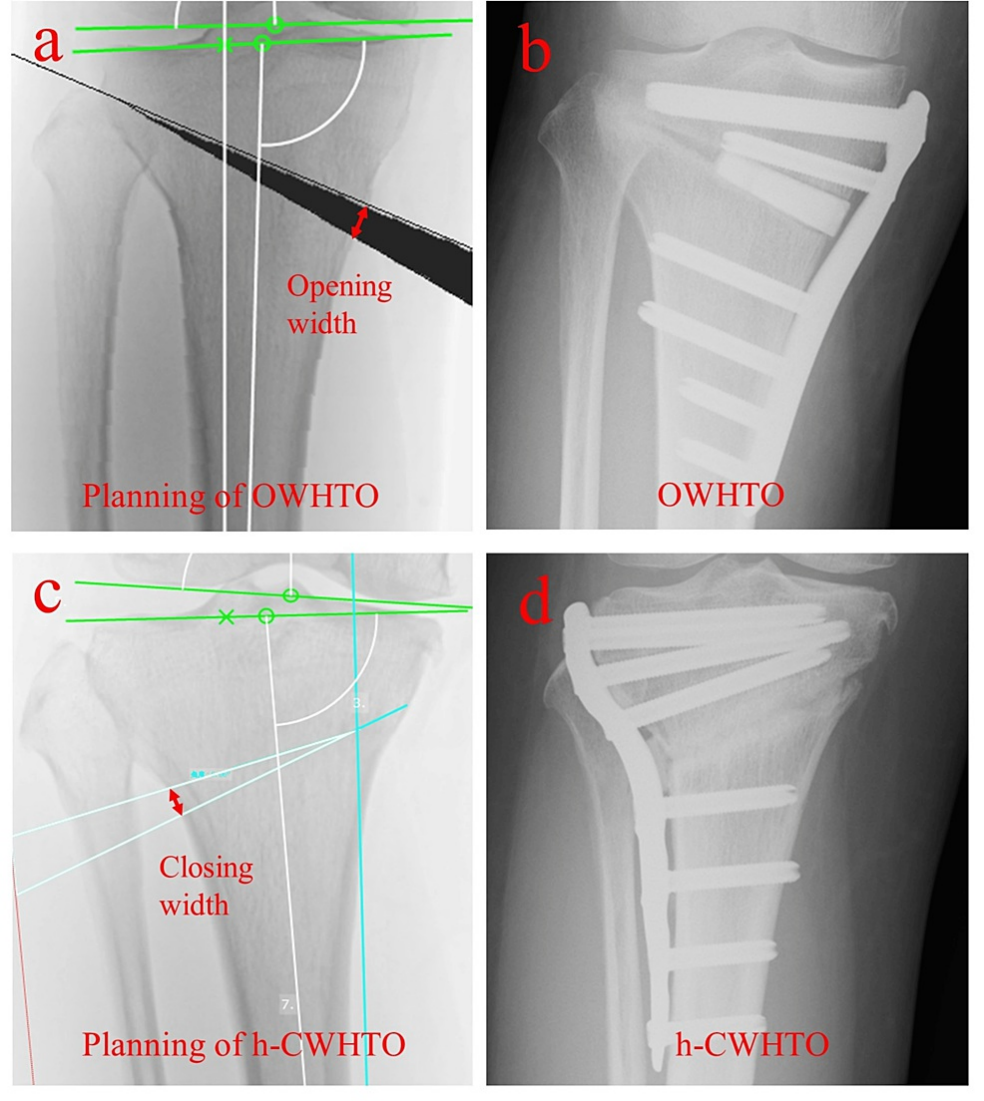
Preoperative planning of OWHTO and h-CWHTO (a) Planning of OWHTO; (b) Postoperative radiograph of the right knee after OWHTO; (c) Planning of h-CWHTO; (d) Postoperative radiograph of the right knee after h-CWHTO OWHTO: Opening wedge high tibial osteotomy; h-CWHTO: hybrid closed wedge high tibial osteotomy.
